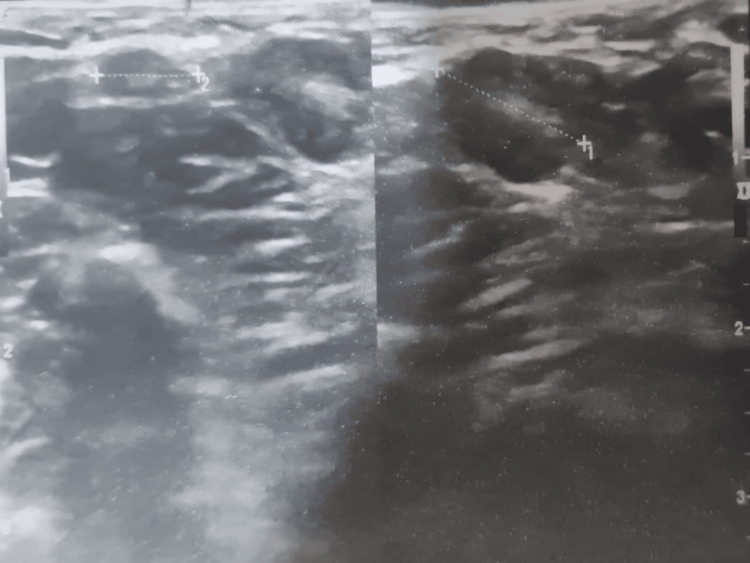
Ultrasound revealed multiloculated, hypoechoic cystic lesions with septa of varying thickness.
